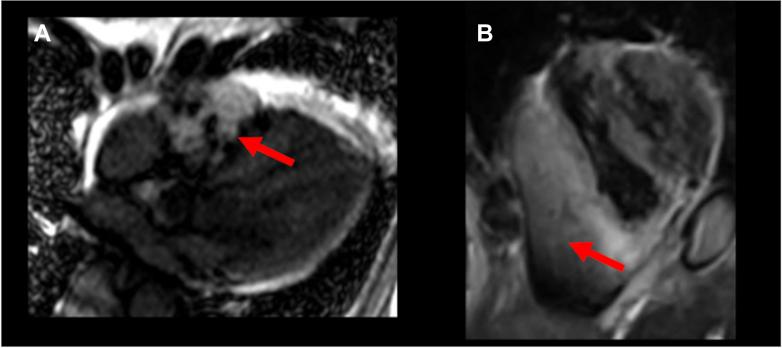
Cardiac Magnetic Resonance The mass (red arrows) shows heterogeneous, indeterminate enhancement on 4-chamber late gadolinium enhancement image (A) and variable intensity on short-axis fat-suppressed T2-weighted image (B).
